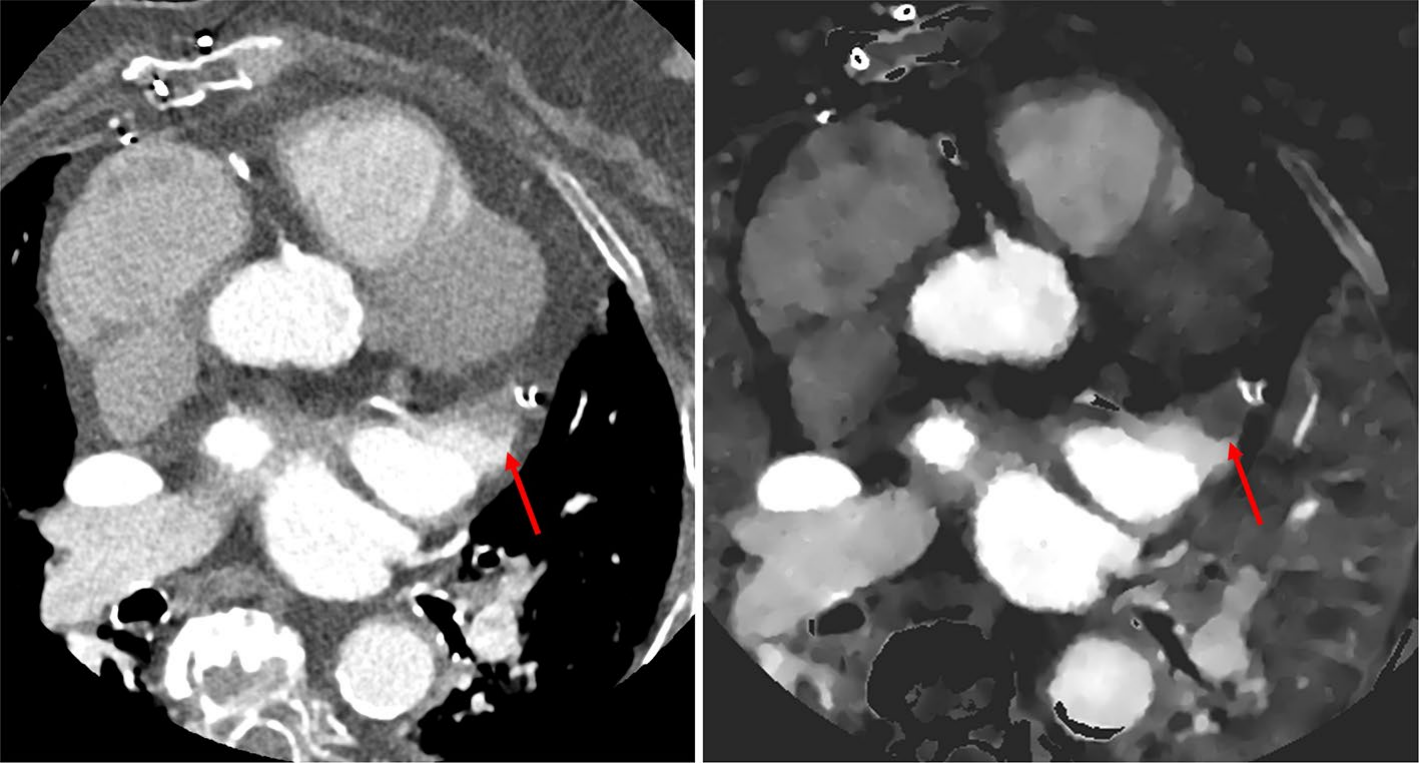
9 mm thrombus in the left atrial appendage, which was missed on conventional cardiac CT images (left). The finding was classified as circulatory stasis. The thrombus was diagnosed on iodine density reconstructions (right).
